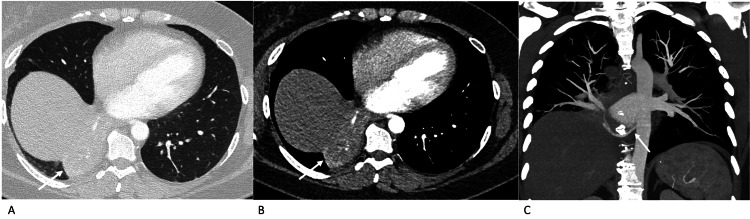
Case 3 - axial contrast-enhanced CT of the thorax Contrast-enhanced CT of the thorax, axial lung window (A), axial mediastinal window (B) and coronal maximum intensity projection (C). Pulmonary sequestration in the right lower lobe is seen as an area of consolidation (arrows in A and B) supplied by an arterial branch arising directly from the descending thoracic aorta (arrow in C).
